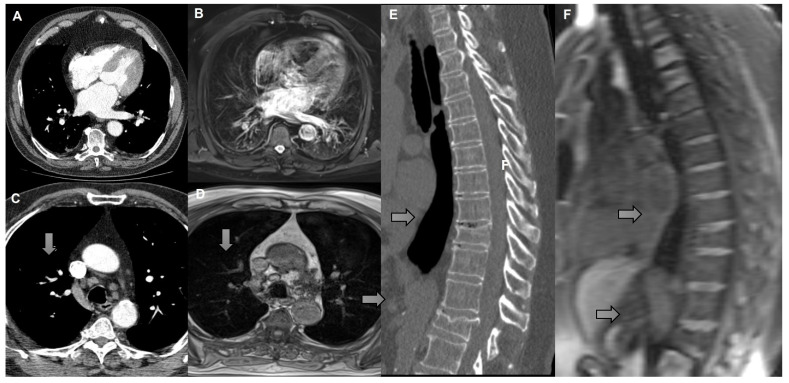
(A) Axial CT scan and (B) T2 with fat saturation MRI demonstrating cardiomegaly (left atrial enlargement). (C) Axial CT scan and (D) T1 with VIBE MRI demonstrating right lower paratracheal lymph node measuring 1.1 cm in short axis (arrow). (E) Sagittal reconstruction CT scan and (F) sagittal reconstruction T1 with VIBE MRI demonstrating vertebral fractures (arrow).
